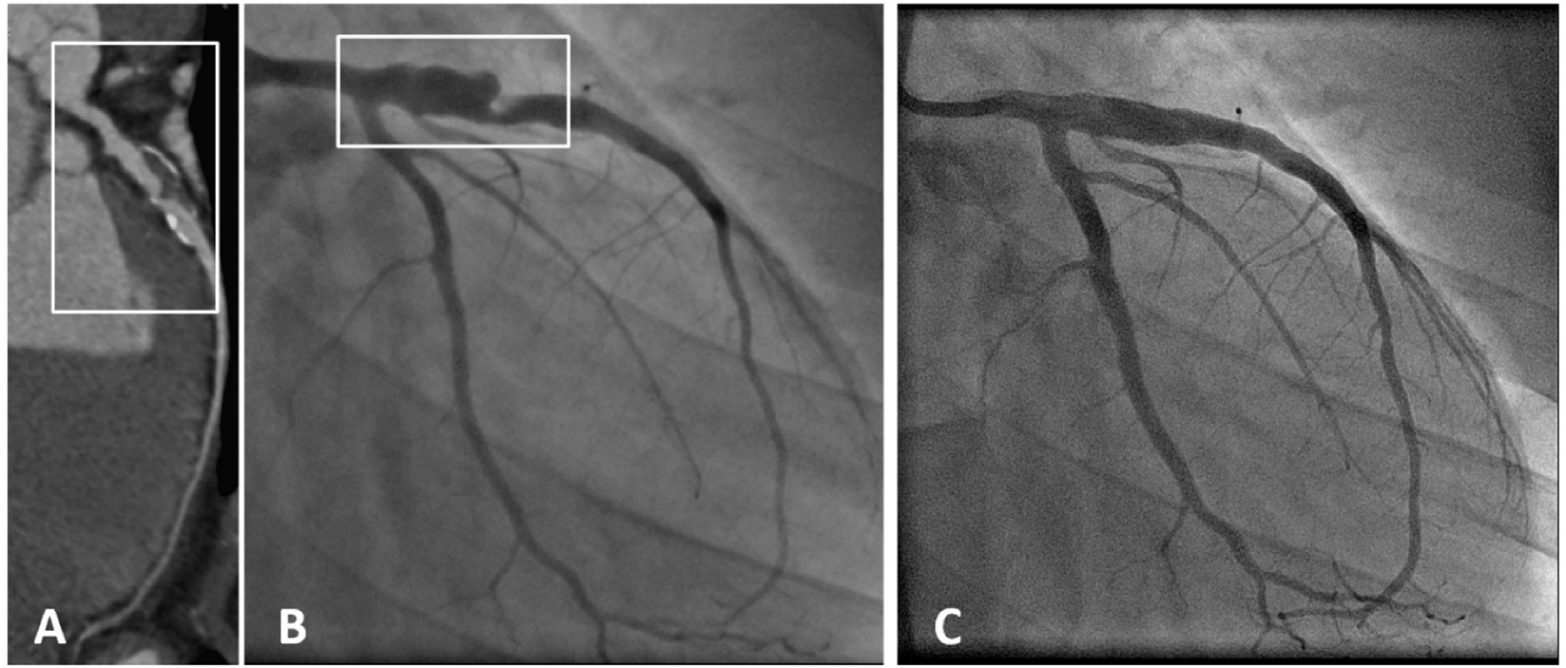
A patient with coronary aneurysm due to Kawasaki disease. (A) Computed tomography angiography showed a large aneurysm (maximum diameter 9 mm, extending for more than 35 mm) of the proximal left anterior descending (LAD) artery with calcific degeneration, large filling defect with thrombus and severe lumen narrowing. (B) Coronary angiography confirmed a 70% stenosis of the LAD artery with an aneurysm immediately distal to the ostium (maximum diameter 9.2 mm × 7.8 mm). (C) Final angiography demonstrating exclusion of the aneurysm using a covered stent [with permission from the report by Di Mario et al. (133)].
